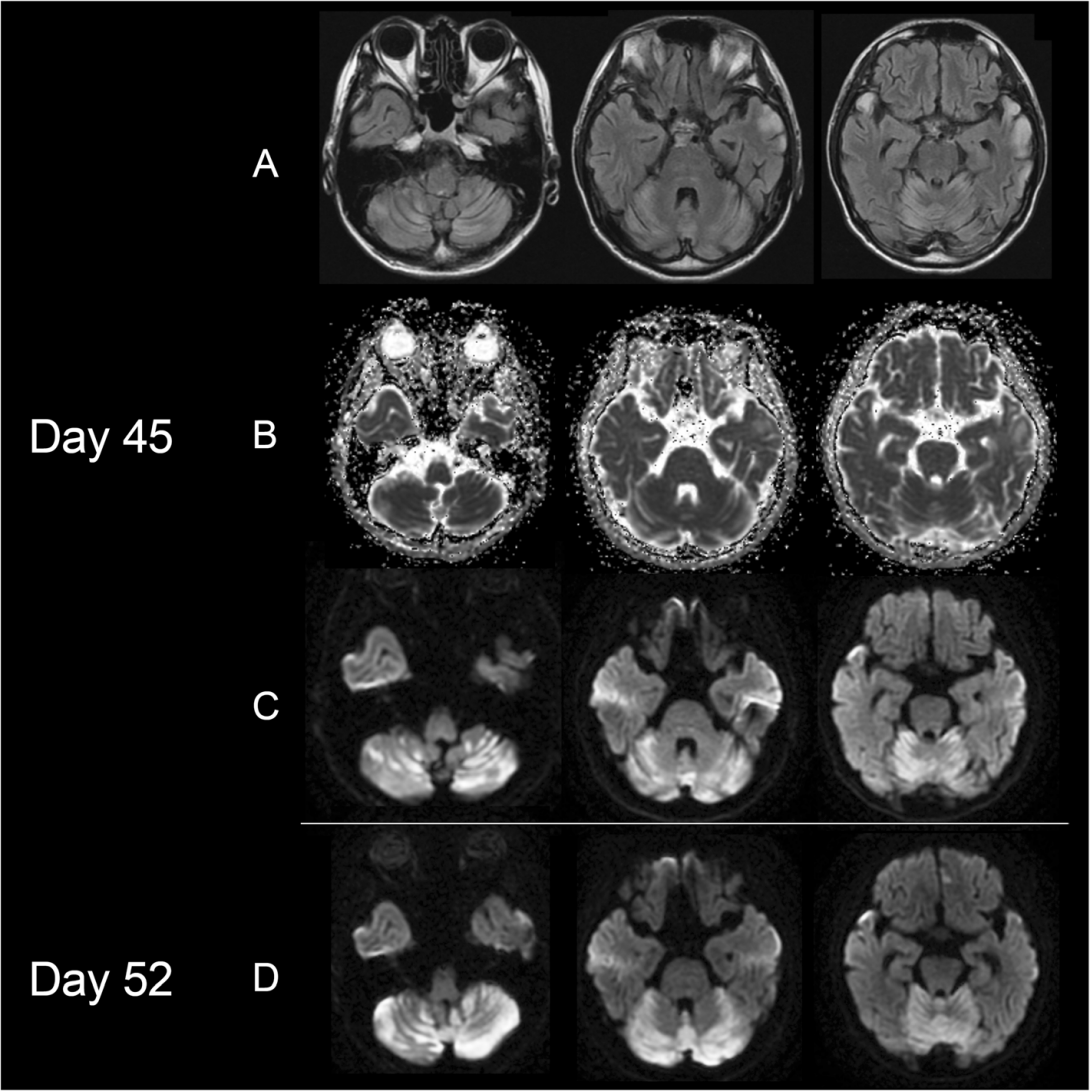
Brain MRIs obtained during the acute stage of cerebellar stroke-like episode. A brain MRI obtained on day 45 (2 days after the onset of dizziness) shows extensive increased DWI/FLAIR signals in the cerebellum, without apparent reduction in ADC values (a-c). Note preferential involvement of the cerebellar folia sparing deep cerebellar nuclei or cerebellar peduncles, and progressive expansion of edematous lesions along the folia on day 52 (d). A: FLAIR: B: ADC map; C and D: DWI
